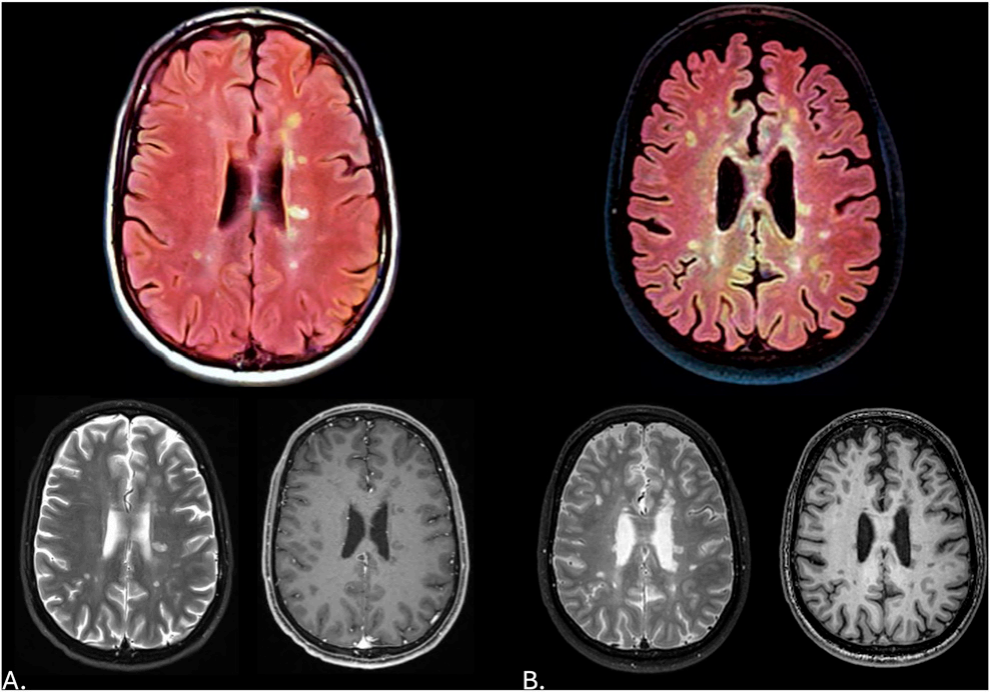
(A) Color transformed axial 3.0 Tesla (T) fluid attenuated inversion recovery (FLAIR) image of the brain with corresponding T2-weighted and post-contrast T1-weighted images at the same level from January 2014. (B) Color transformed axial 3.0 T FLAIR image with corresponding T2-weighted and T1-weighted images at the same level from June 2024. Note the changes in lesion number and size along with the generalized reduction in brain volume.
